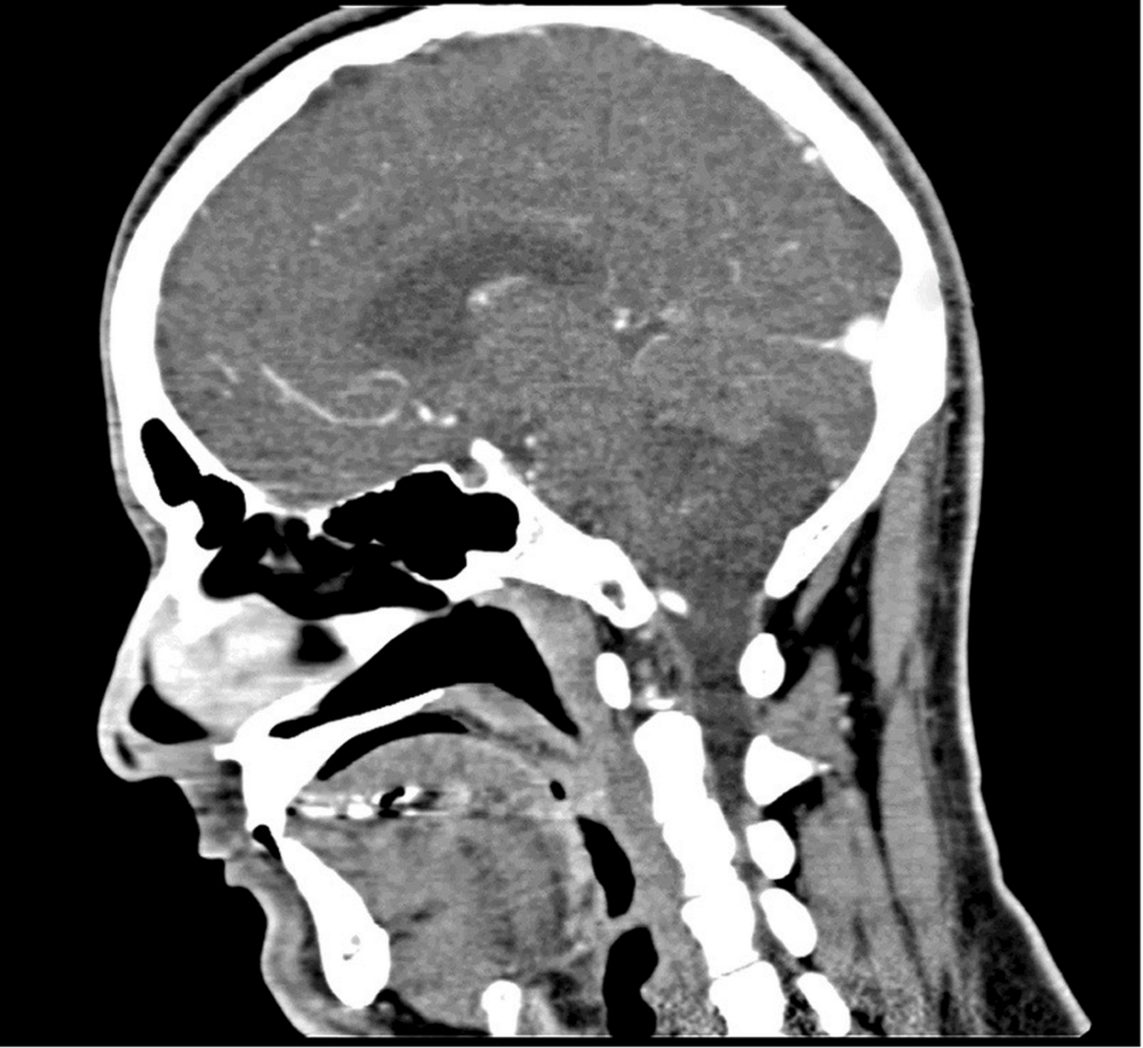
Preoperative sagittal CT scan showing a posterior fossa mass involving the cerebellar region.
